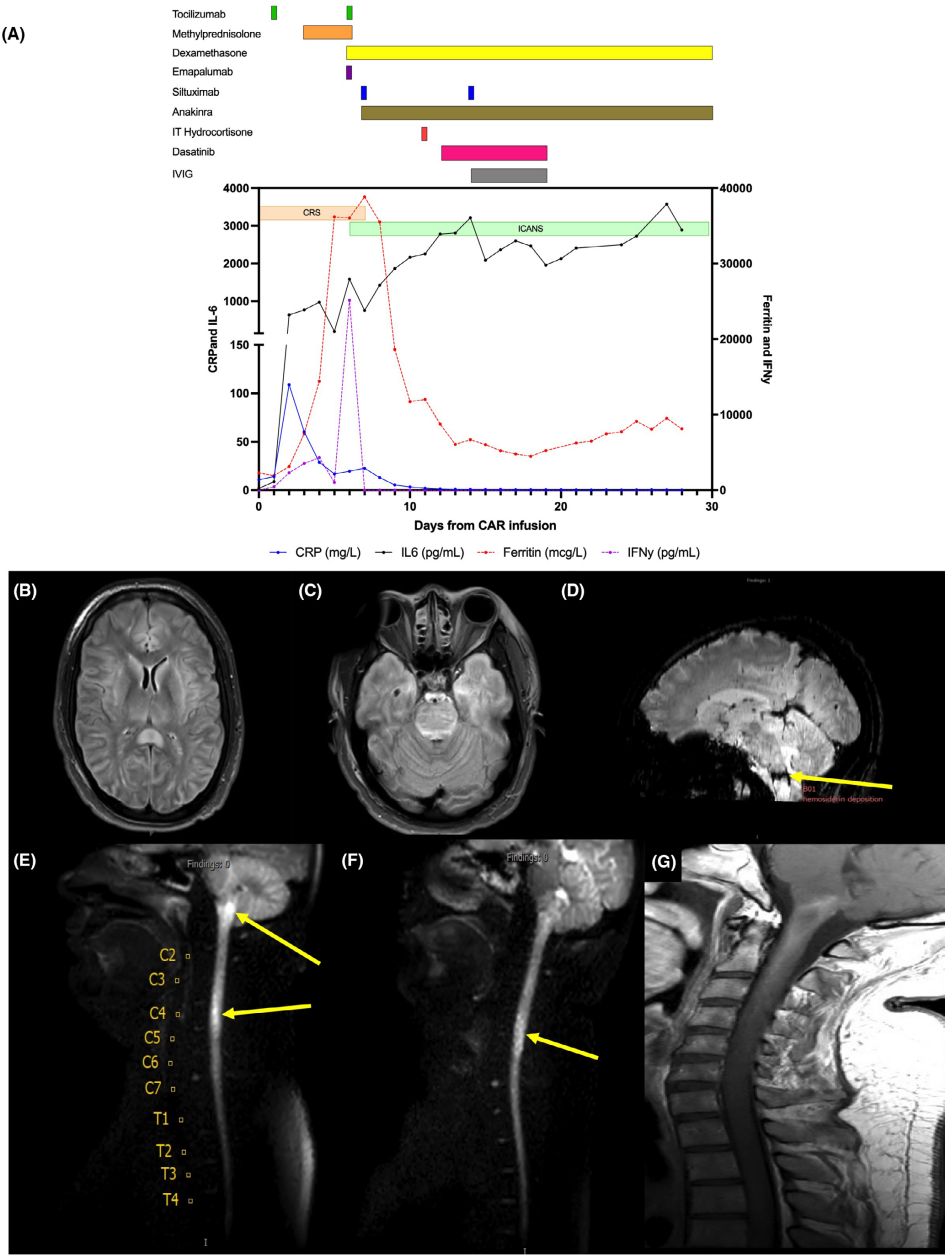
Clinical course and central nervous system imaging. (A) Timeline displaying toxicity course as days from CAR T‐cell infusion on the *x*‐axis (day 0 = infusion). Onset and duration of CRS and ICANS are shown by the shaded bars. Graphical quantification of inflammatory markers including plasma C‐reactive protein (CRP), interleukin (IL)‐6, ferritin and interferon (IFN)‐γ is also shown. Above the graph, pharmacological interventions targeted for toxicity management are displayed; bars represent the timing of respective interventions. (B, C) MRI brain on day +7 showing diffuse cerebral oedema throughout the (B) basal ganglia, insular cortex and cerebrum as well as the (C) pons. (D, E) MRI brain and spine on day +10 showing (D) signal abnormality and haemorrhage in the cervico‐medullary junction suggesting spinal cord ischaemia or infarction as well as (E) diffusion restriction of the spinal cord at the cervico‐medullary junction and at the C4–C5 level. (F, G) MRI spine on day +21 showing (F) persistent restricted diffusion in the cervical cord as well as (G) sagittal T1 precontrast demonstration of the presence of methaemoglobin throughout the spinal cord, suggesting subacute spinal cord haemorrhage. CAR, chimeric antigen receptor; CRS, cytokine release syndrome; ICANS, immune effector cell‐associated neurotoxicity syndrome; MRI, magnetic resonance imaging.
